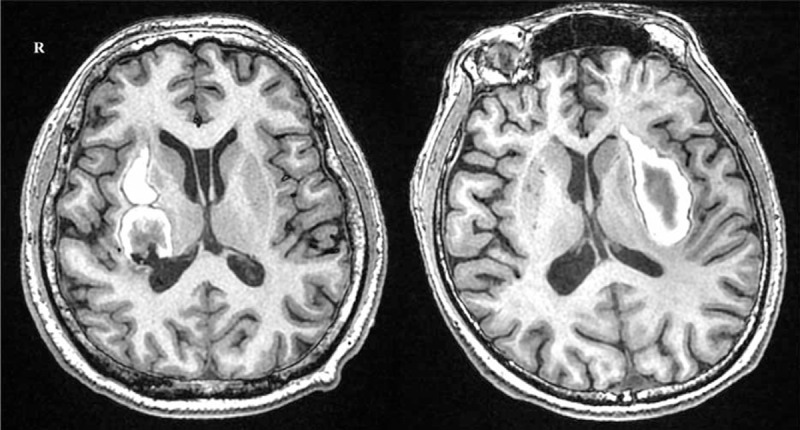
3D T1-weighted images of the primary lesion (left, Subject 1; right, Subject 2).
